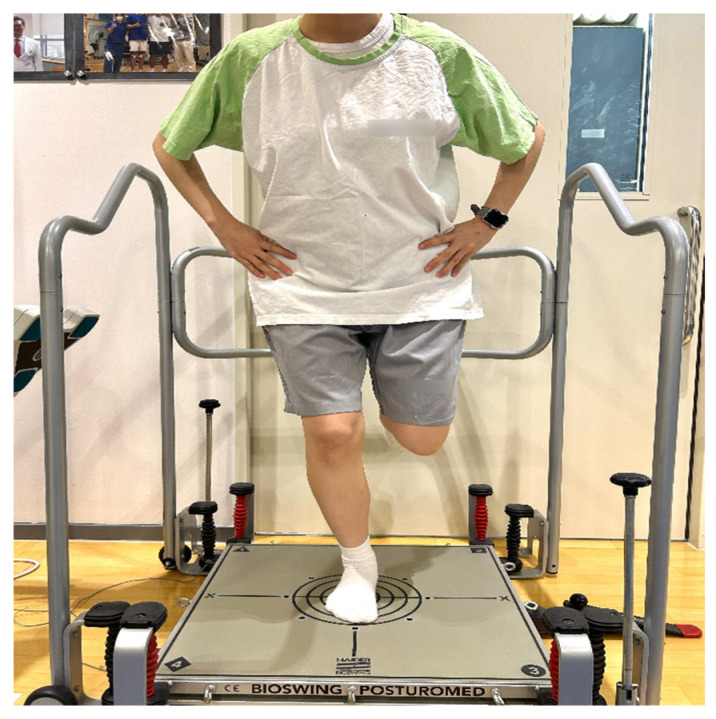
Single-leg stance assessment on the POSTUROMED platform with sway path recorded using the CMS-10 ultrasound-based motion analysis system (Zebris Medical GmbH, Isny im Allgäu, Germany).
